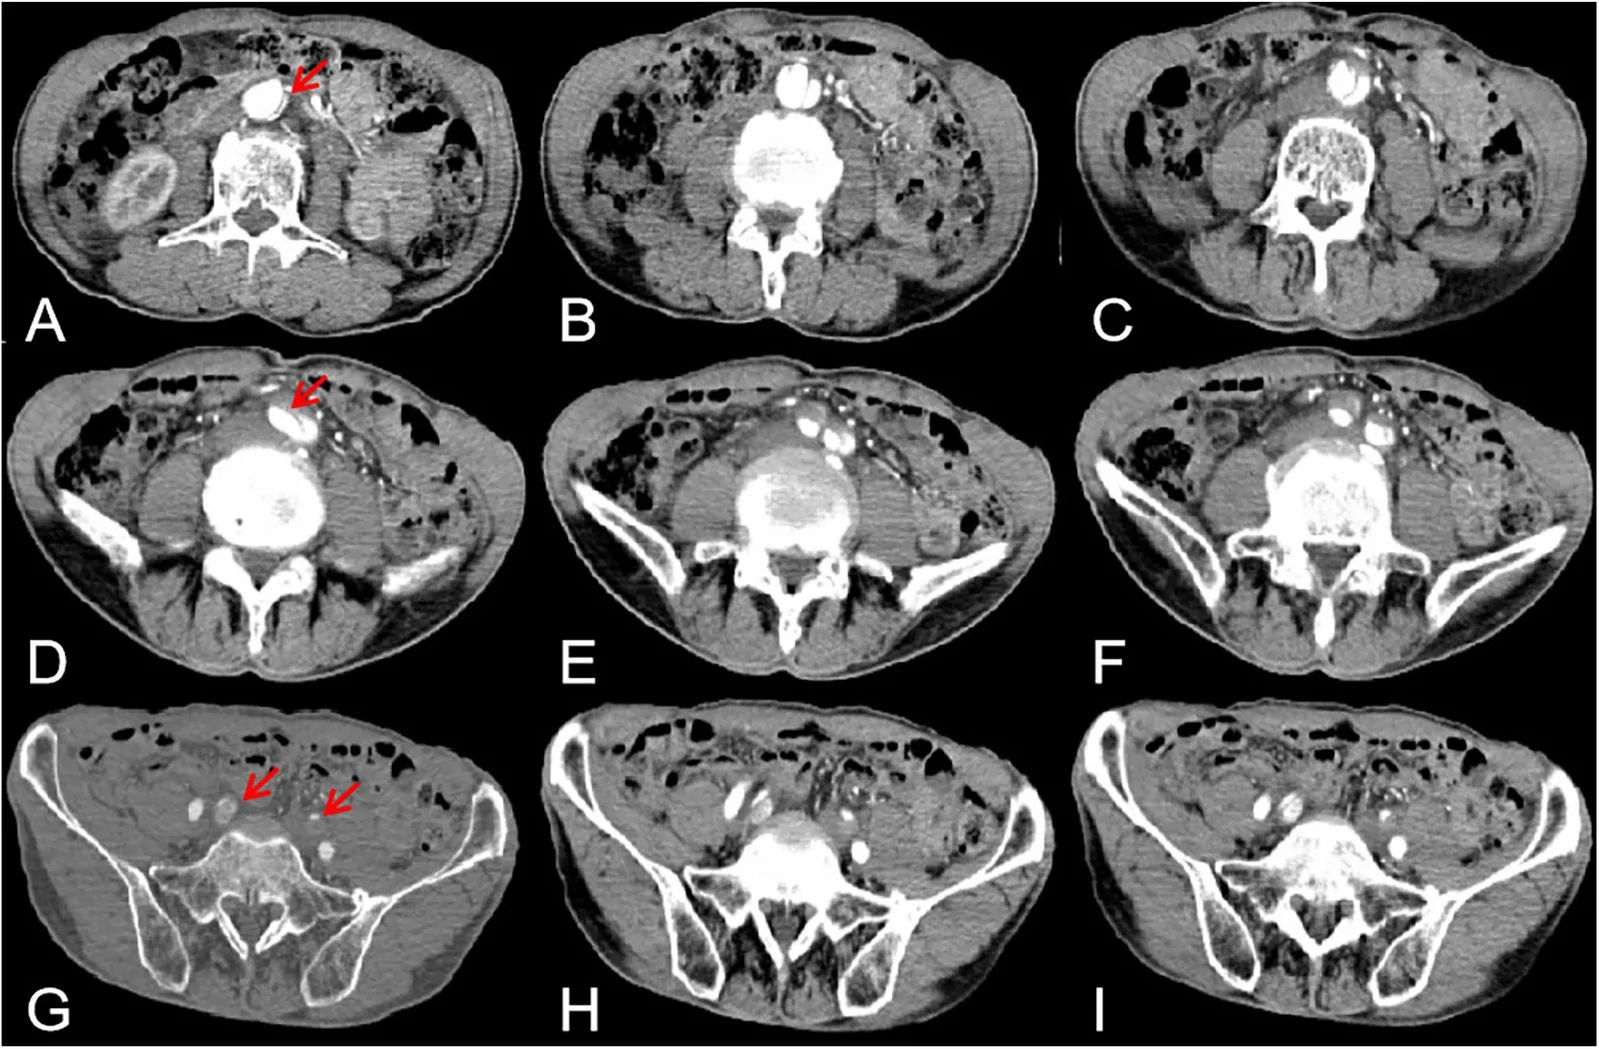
One–month posT,discharge surveillance CTA (A–C) distal abdominal aorta level: stable appearance of the dissection flap (arrow) with organized intramural hematoma. (D–F) Aortoiliac bifurcation level: The dissection (arrow) remains stable with no significant interval change. (G–I) Iliac artery level: The iliac artery dissection (arrows) shows complete stability with further hematoma resolution.
